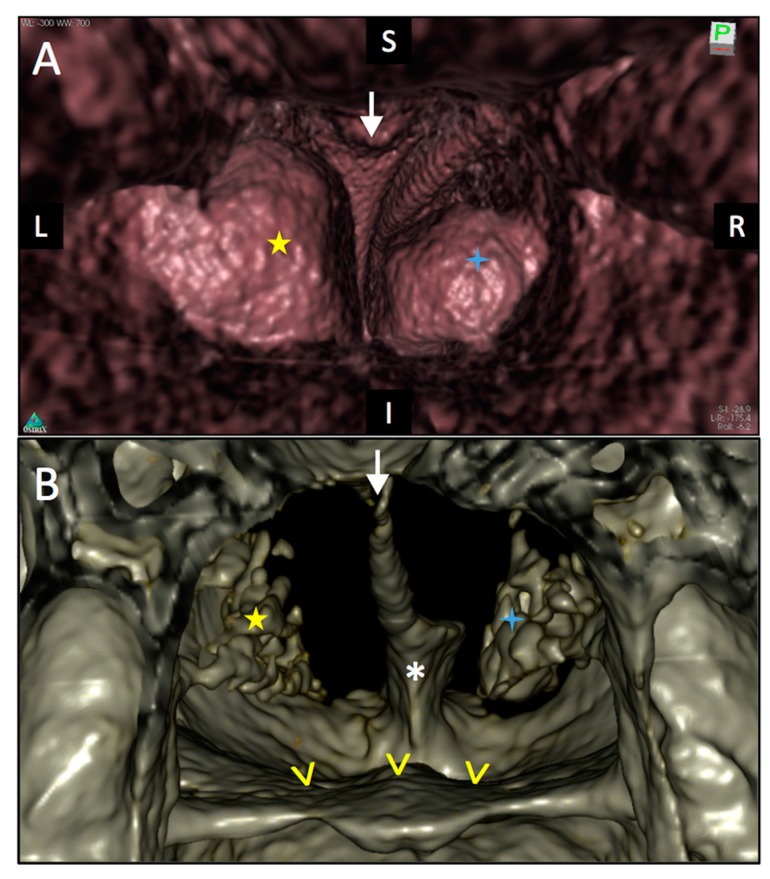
3D virtual endoscopic reconstruction (A) and 3D bone reconstruction (B) of the CBCT data set. This posterior view of the choanae shows the absence of separation between the left (L) and right (R) nasal fossae in their posterior part. Yellow star: lower left turbinate; Blue star: right lower turbinate; White arrow: Reversed triangular shape formed by the poster and inferior edges of perpendicular plate of the ethmoid bone. Yellow arrowheads: floor of the nasal fossae. White asterisk: Palatine process of the maxillary bone.
